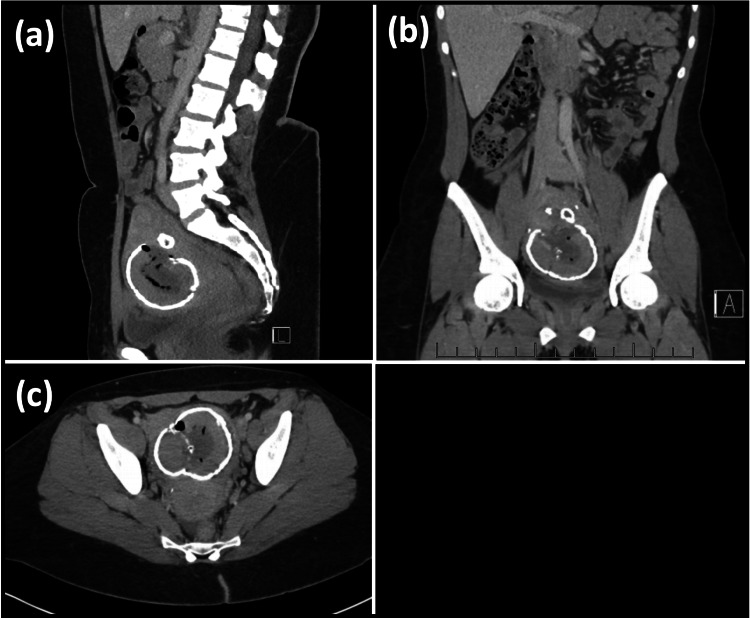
CT of the abdomen/pelvis with contrast showing calcified fibroid measuring 6.8×7.2×6.9 cm: (a) sagittal view, (b) coronal view, and (c) axial view CT: computed tomography
